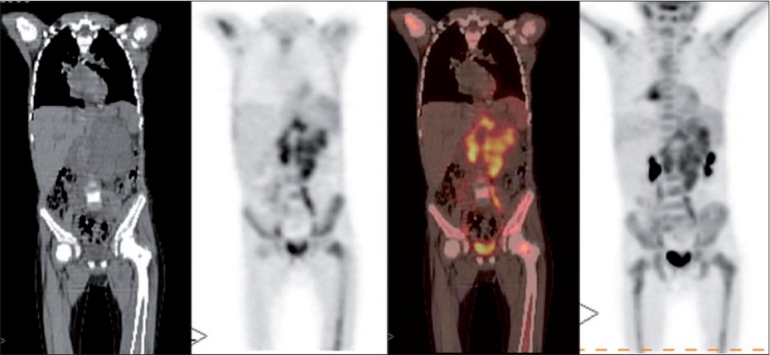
CT, PET, PET-CT and maximum intensity projection images (Left-Right) showing the presence of extensive disease in the abdomen and diffuse marrow metastasis
